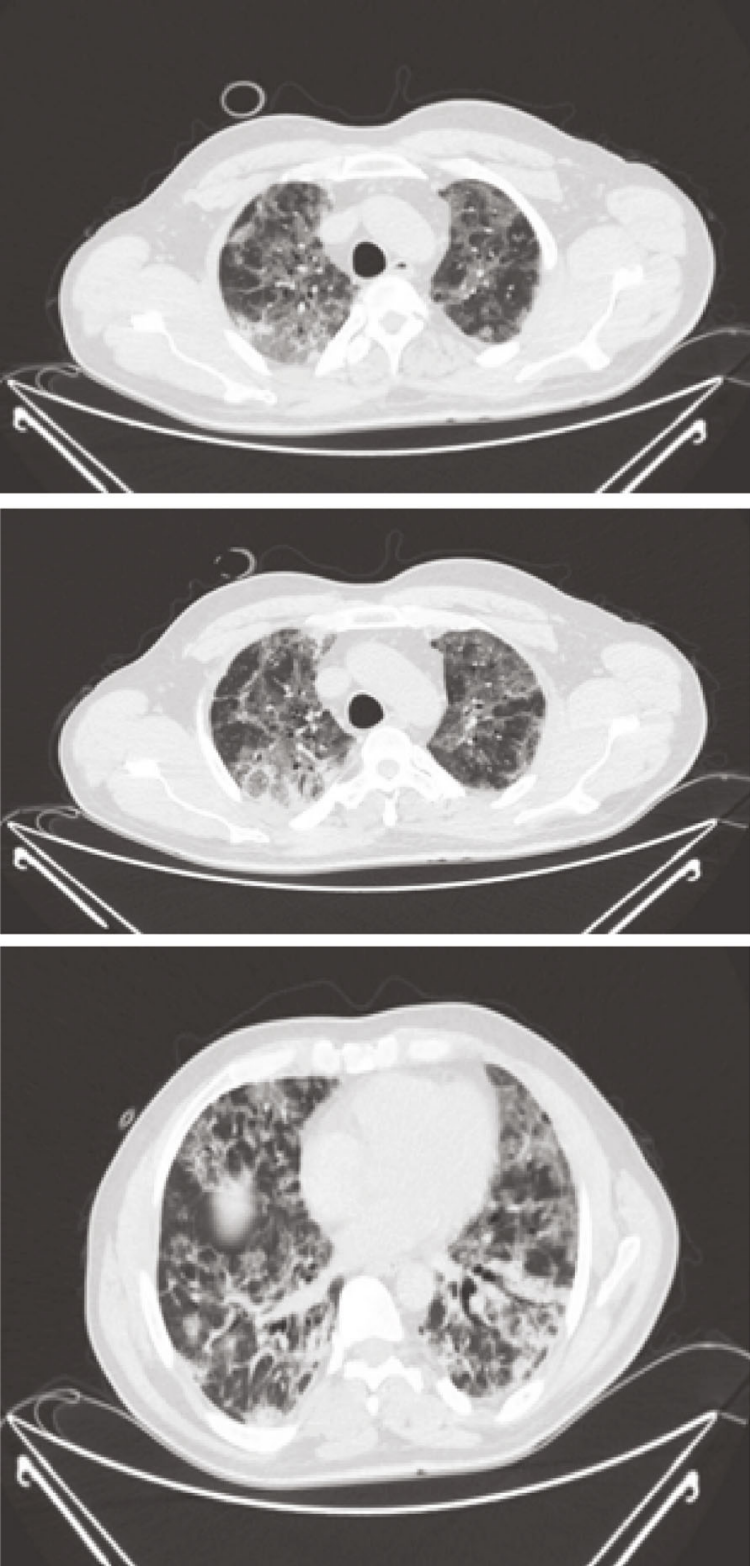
Chest CT shows pathology typical for SARS-COV-2.
